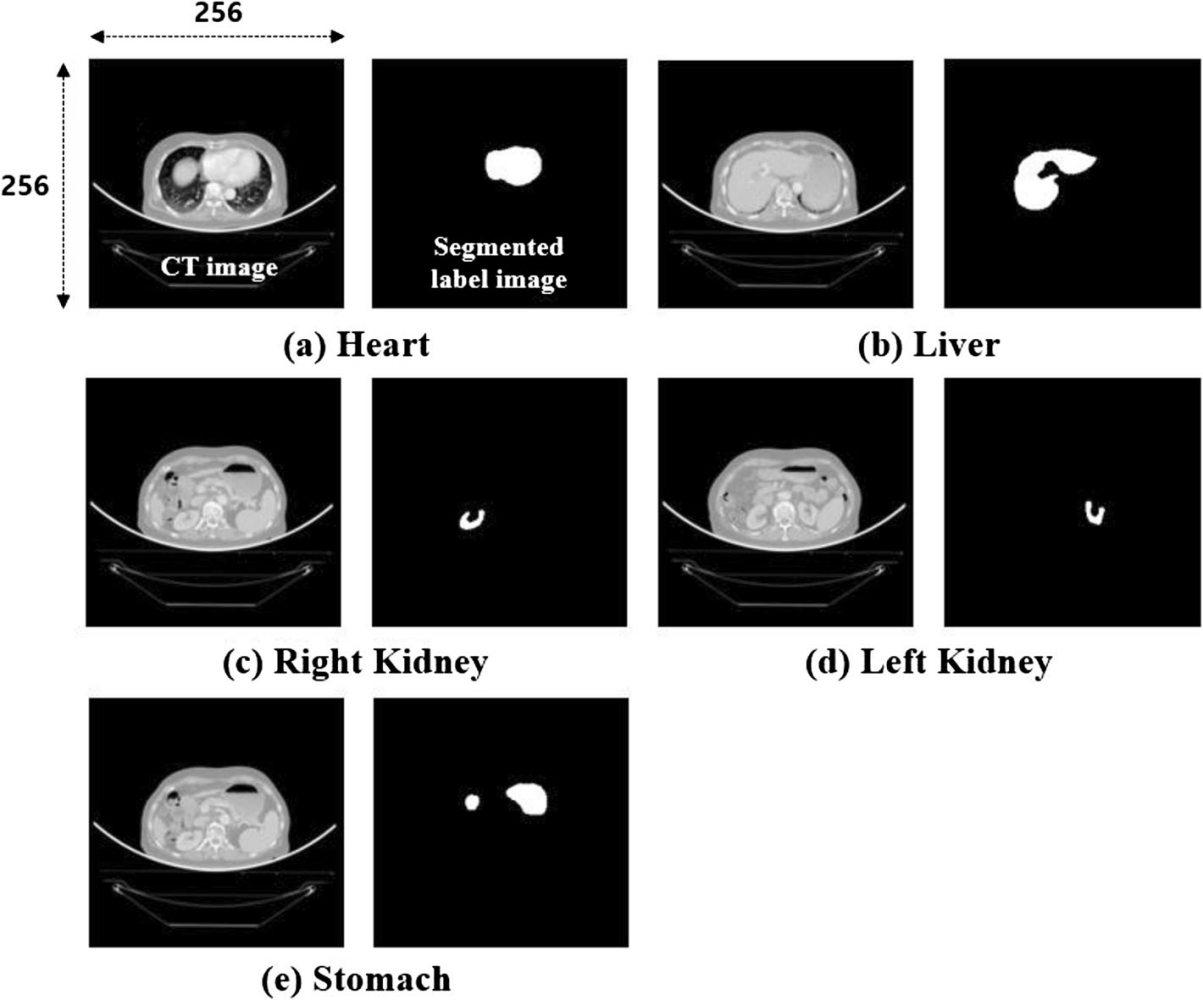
Grayscale CT and segmented label images of the (a) heart (H), b liver (L), c right kidney (RK), d left kidney (LK), and e stomach (S) used for DCNN model learning
